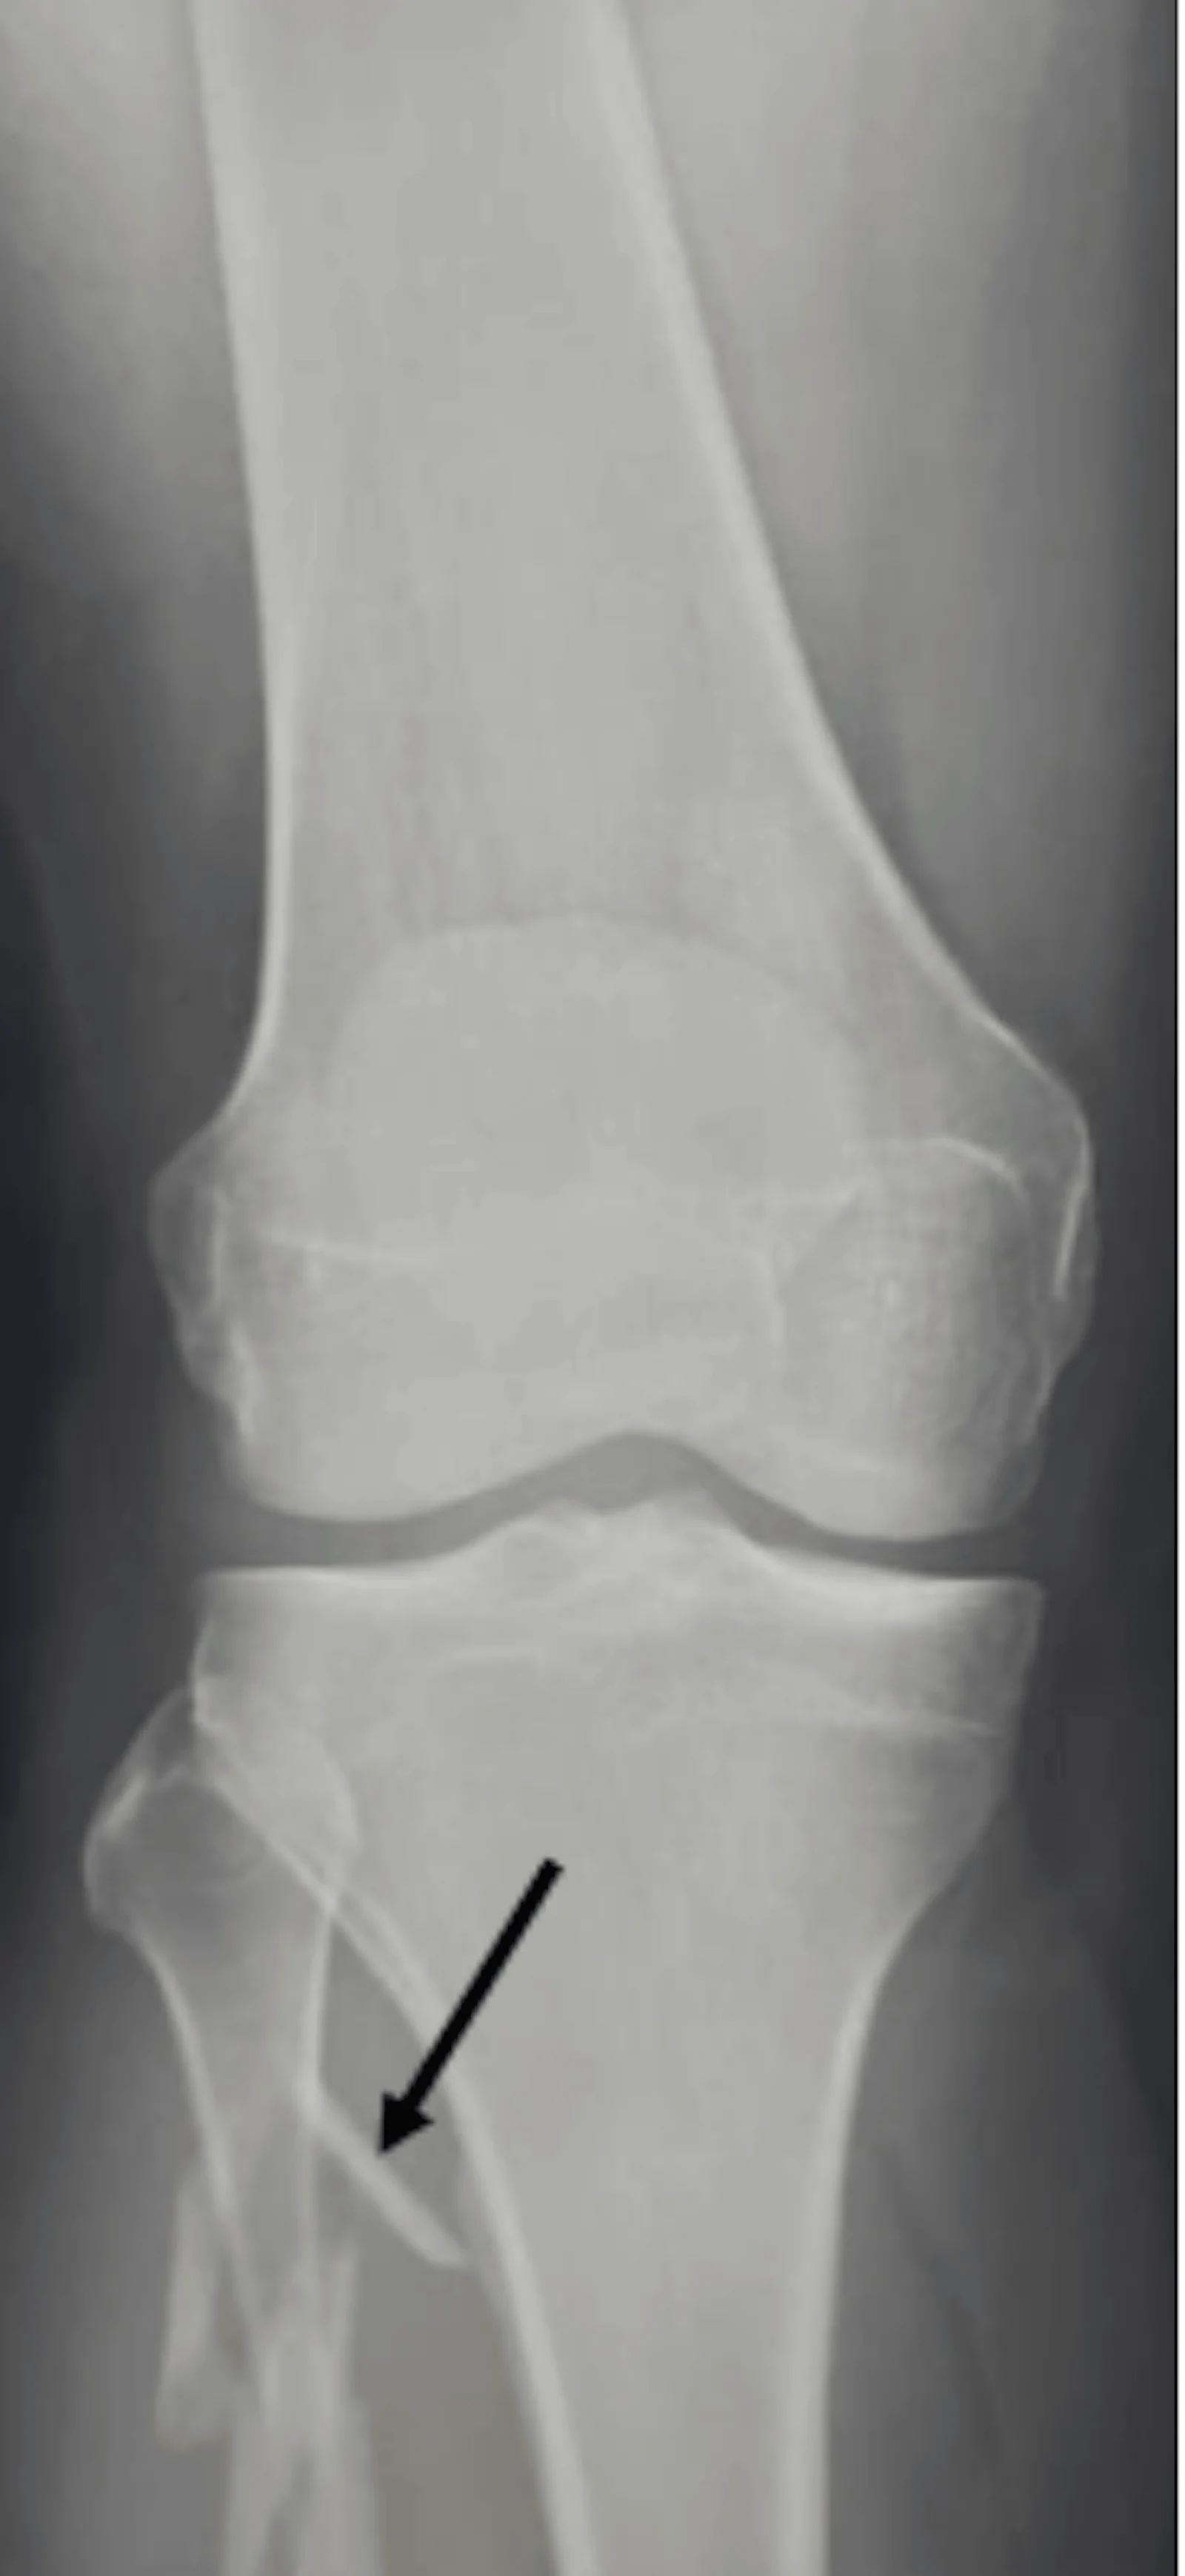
Anteroposterior radiograph of the right knee showing the sharp fragment of the proximal fibula fracture causing injury to the popliteal artery (arrow).
